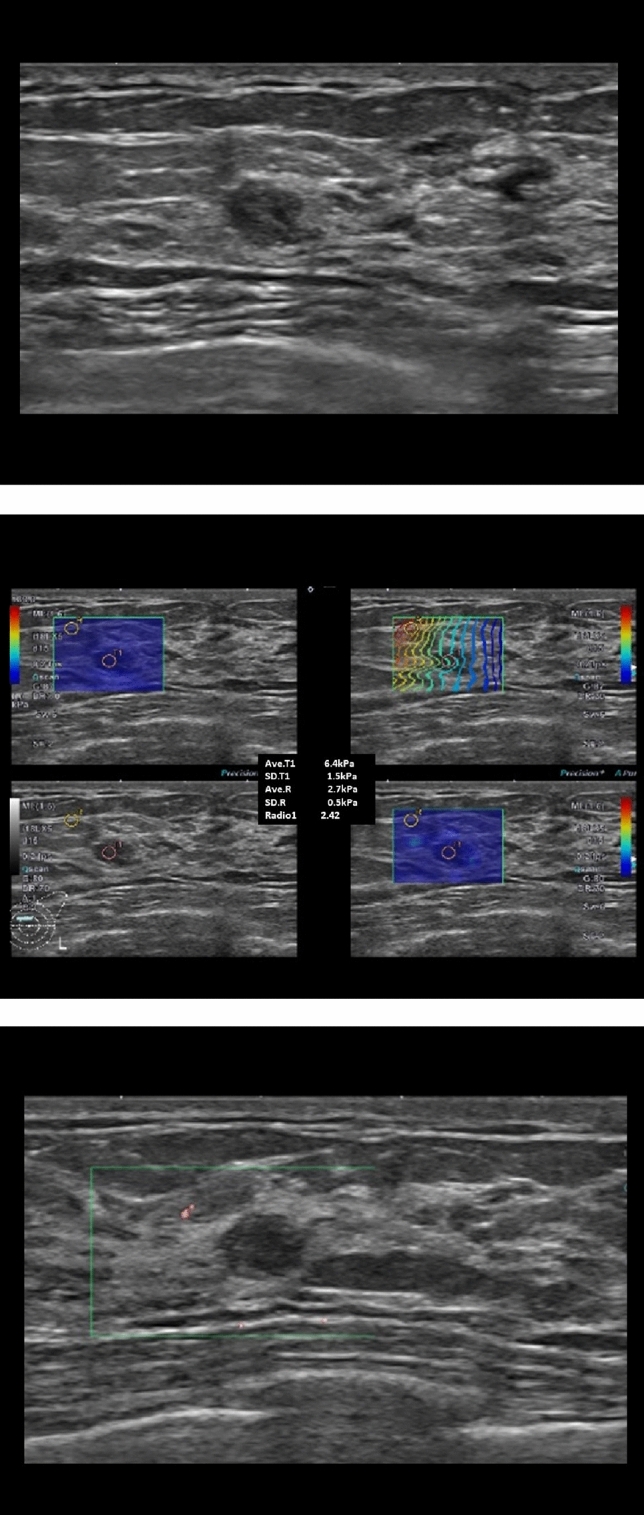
A 32-year-old female patient with fibroadenoma. (A) B-mode ultrasound showed a 0.6 cm-sized, mass categorized as C4a. (B) The SWEmax and SWE ratio were below the cutoff value as as SWEmax 6.4 kPa and SWEratio 2.42. (C) The vascular index of the SMI was 0%. The combined score was 2, indicating that the lesion was considered benign lesion. The pathologic diagnosis was fibroadenoma.
